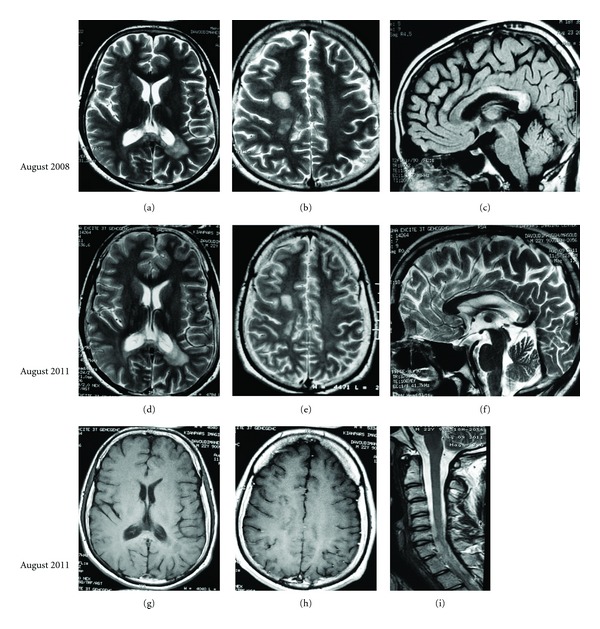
August 2008 (upper row): axial T2WI ((a) and (b)) and sagittal FLAIR (c) and August 2011 (middle row) axial T2WI ((d), (e), and (f)) demonstrate sequelae of lesions as multifocal high T2 signal intensities in right centrum semiovale area and peri-left-occipital horn white matter, involving also splenium and posterior body of the corpus callosum which were stable and the peripheral vasogenic edema is gone. August 2011 (lower row) postcontrast axial T1 W images ((g) and (h)) and sagittal cervical spine T2 WI (i), no enhancement in lesion or abnormal signal intensity in cervical cord were seen.
